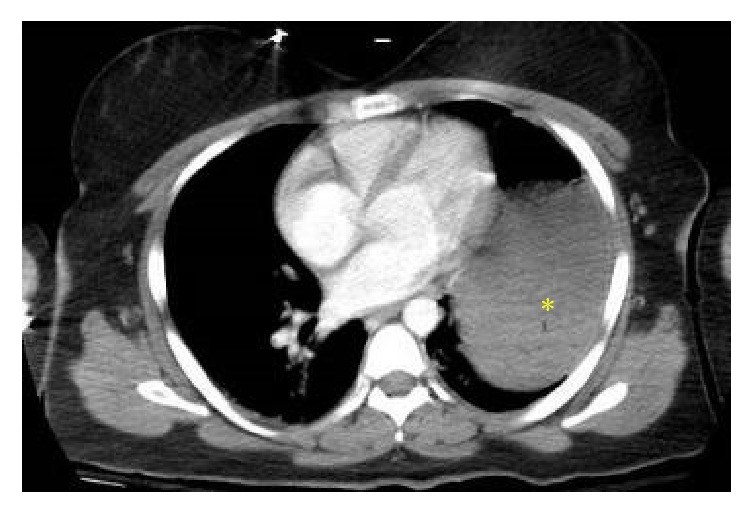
Postcontrast (intravenous) axial CT image confirms a fluid-filled viscus (yellow asterisk) in the lower left thoracic cavity.
